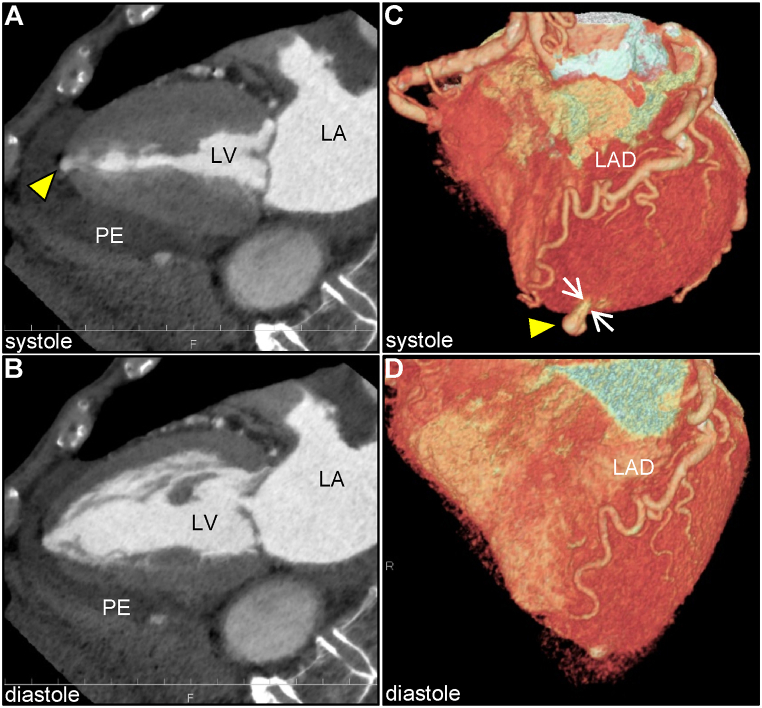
Cardiac computed tomography angiography image of left ventricular apical outpouching. Two-chamber view during systole (A) and diastole (B). Volume rendering image during systole (C) and diastole (D). It is notable that the apical outpouching with a narrow neck (arrows) of the LV caused significant protrusion during systole (arrowhead), and a moderate amount of pericardial effusion surrounding the LV. LA, left atrium; LAD, left ascending artery; LV, left ventricle; PE, pericardial effusion.
